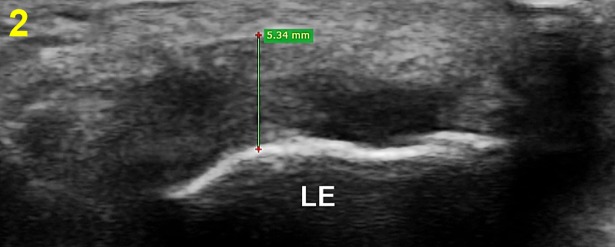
Transverse ultrasound view demonstrating thickness (mm) measurement of the common extensor tendon. LE: lateral epicondyle, R: radius.
